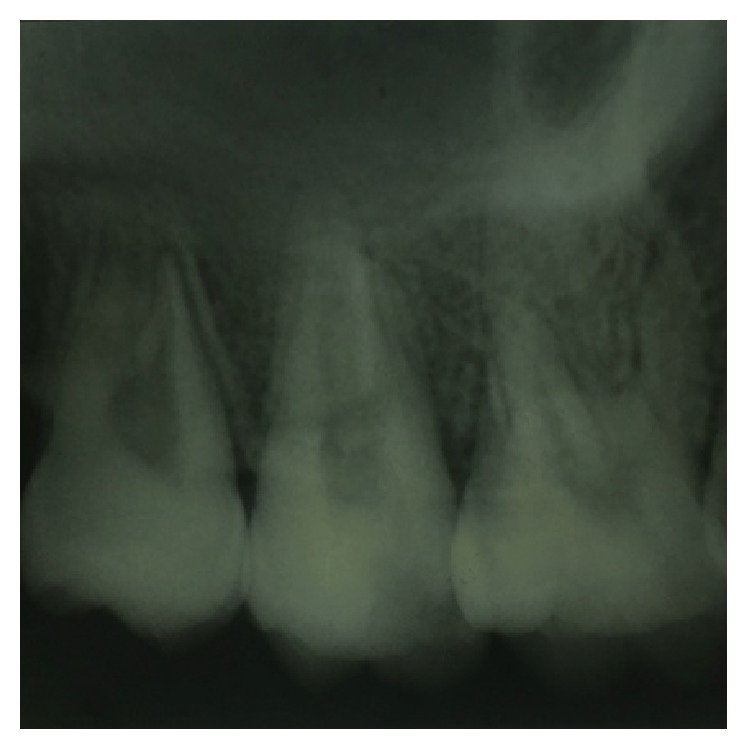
Preoperative radiograph of 27.
